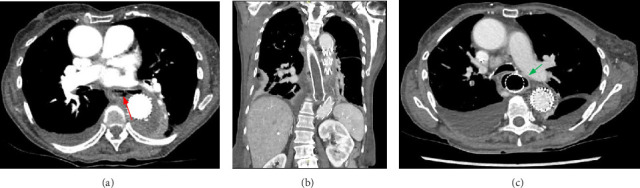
(a) Computed tomography (CT) images showing aorto-oesophageal fistula post repeat TEVAR (red arrow). (b-c) Images showing persisting oesophageal defect after oesophageal stent insertion (green arrow).
